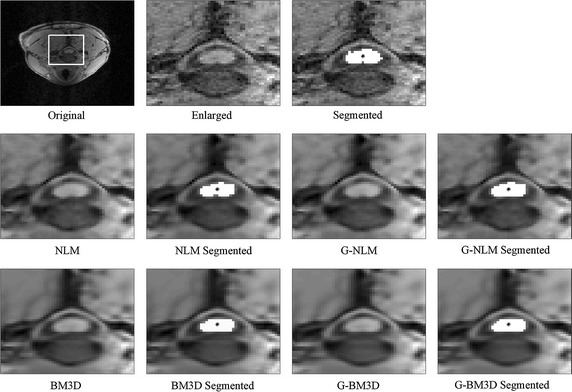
Denoising results for the real MRI dataset of the spinal cord. From *top* to *bottom*: original volume, denoised results and corresponding segmented results using NLM and the proposed global framework, denoised results and corresponding segmented results using BM3D and the proposed global framework
